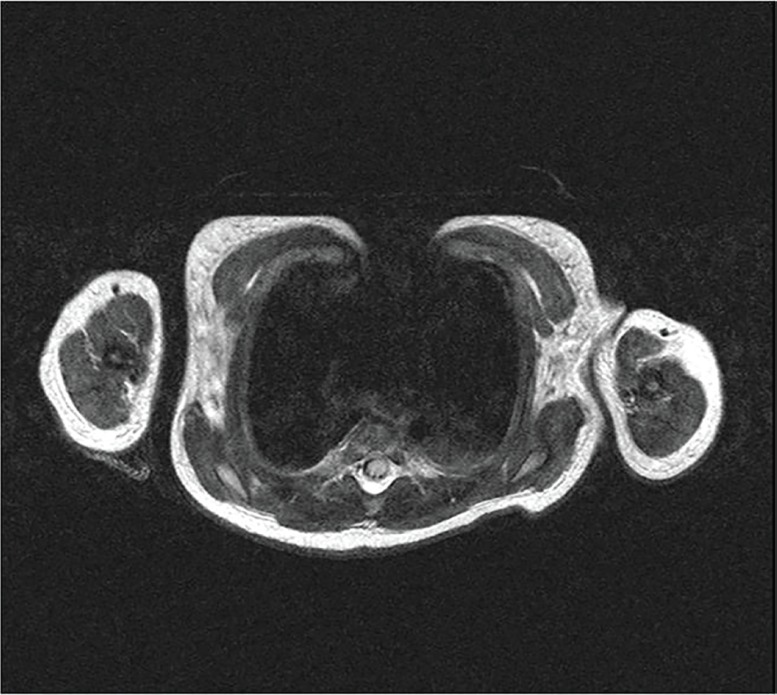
MRI scan of chest – Axial view. Note the anterior chest wall defect
